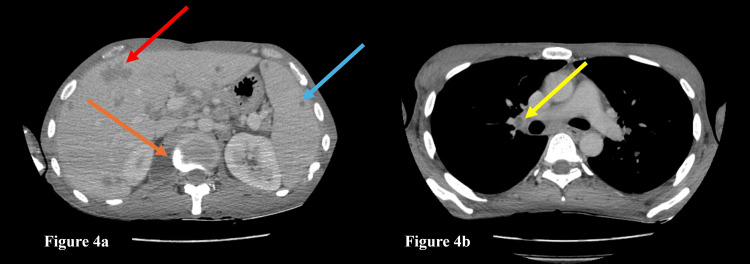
Contrast-enhanced CT (CECT) of the chest and abdomen a: The axial section of the CECT abdomen shows multiple hepatic lesions (red arrow), splenic lesions (blue arrow), and a paravertebral abscess (orange arrow); b: The axial section of the CECT chest shows a necrotic right hilar lymph node (yellow arrow).
